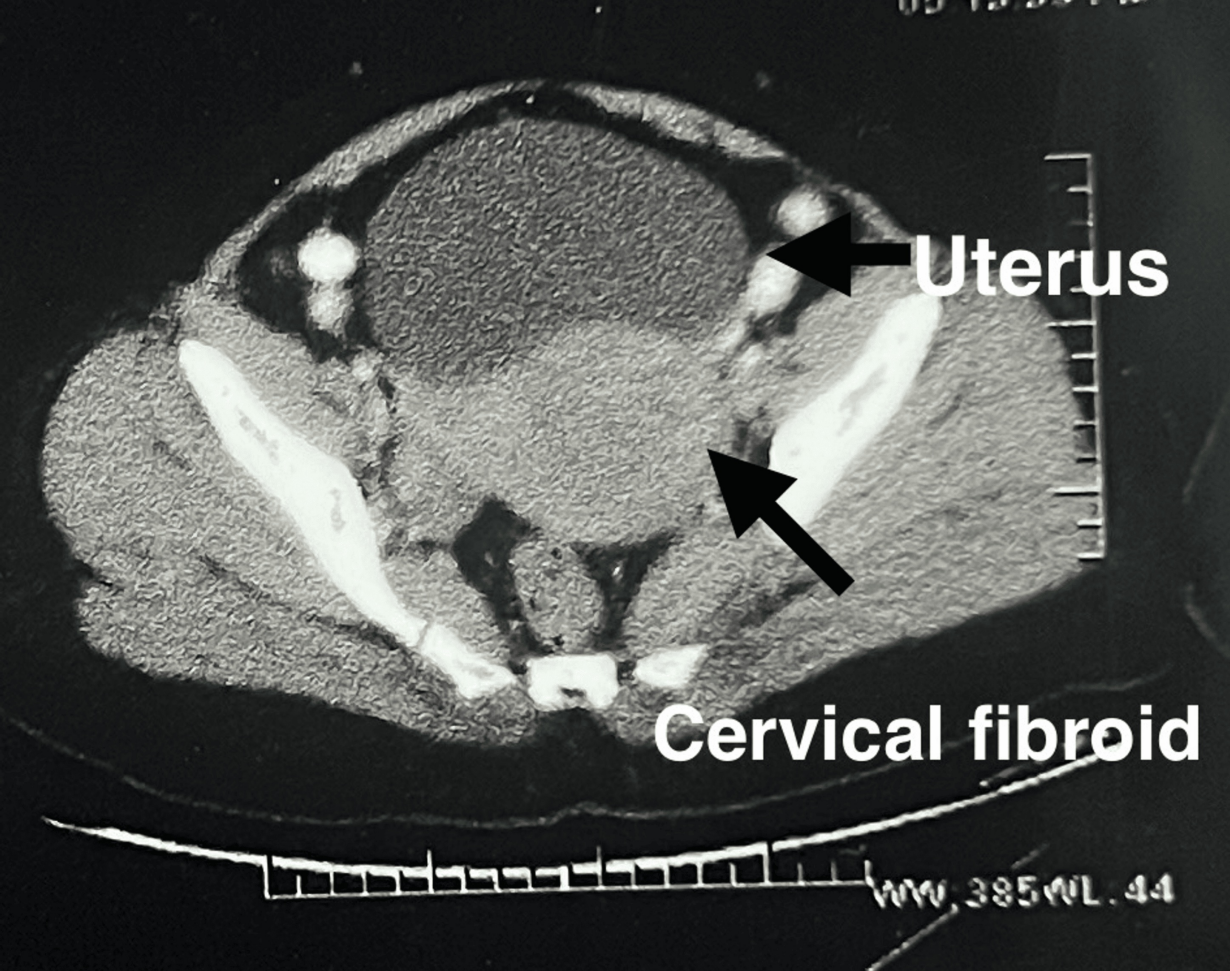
CT of the abdomen and pelvis with contrast revealed a cervical fibroid in the left lateral wall extending till the cervical region of the uterus, measuring 8.4 x 7.3 cm
